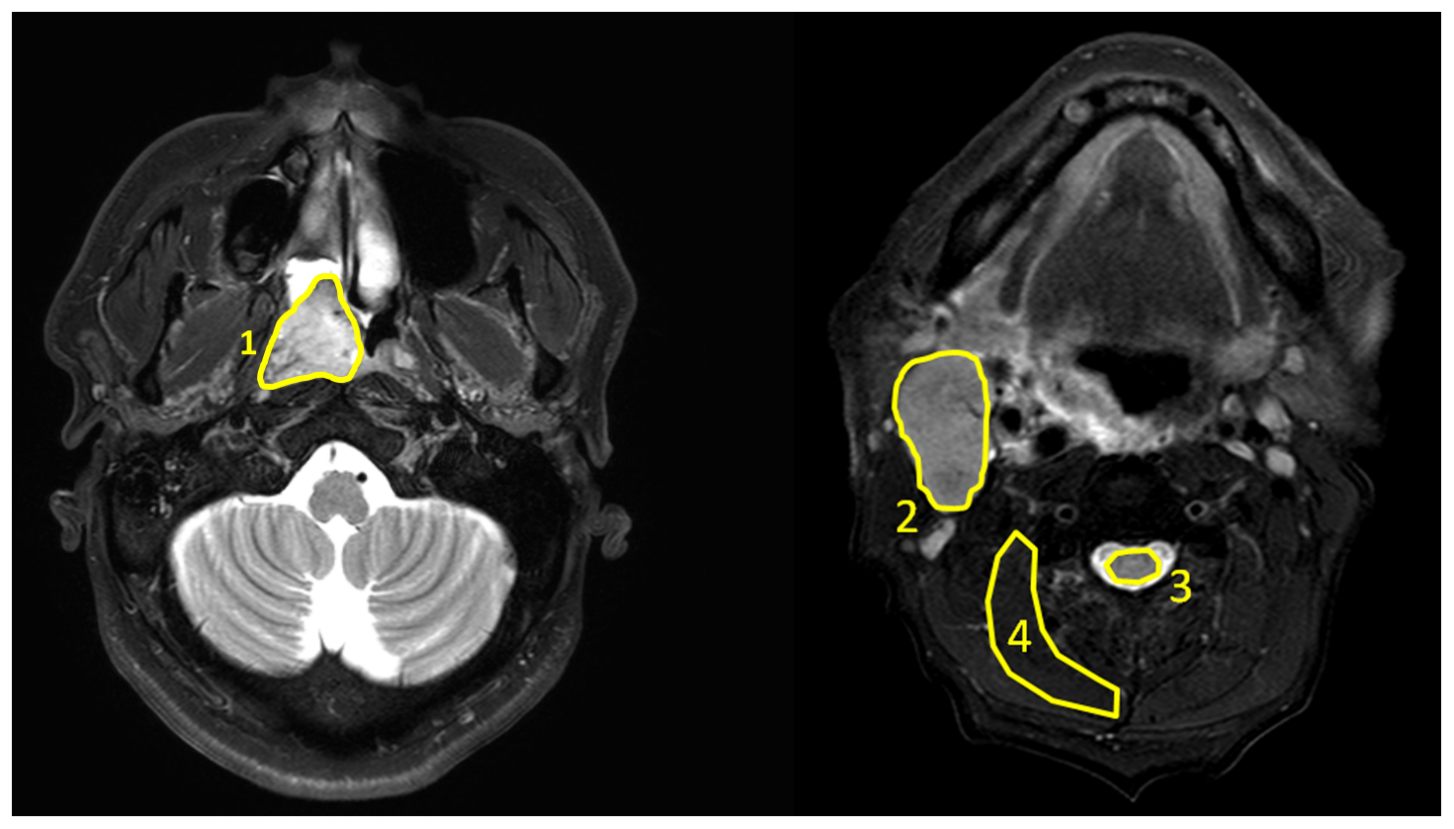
T2-weighted fat-suppressed fast-spin-echo anatomical images. Regions of interest (ROIs) were drawn on primary tumors of nasopharyngeal carcinoma (NPC), metastatic node, spinal cord and muscle and labeled as one to four respectively.
